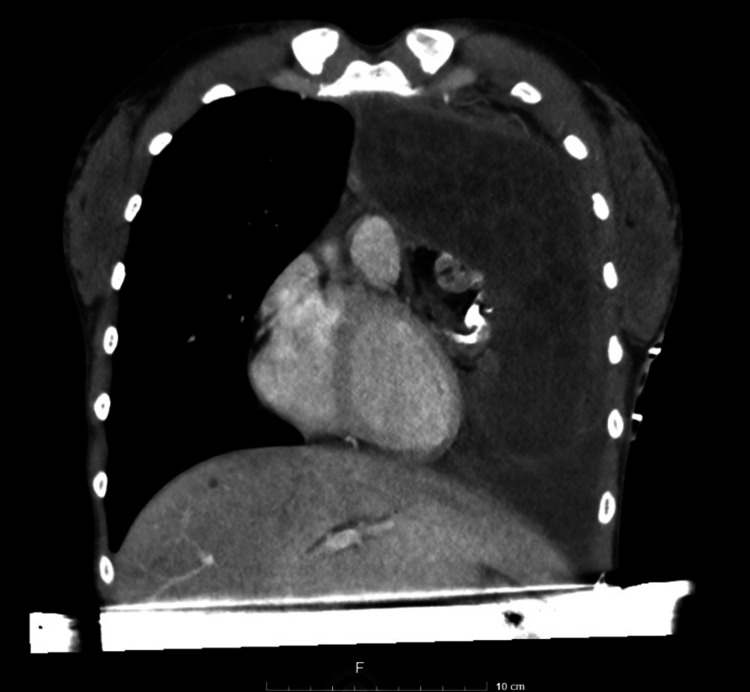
Preoperative chest computed tomography, coronal view showing a large anterior mediastinal mass expanding to the left chest.
